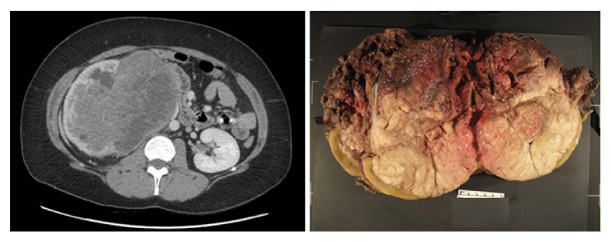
CT imaging shows a heterogeneous partially calcified enhancing solid mass of the right kidney and gross findings show a necrotic and friable soft tissue mass involving the distal ureteric margin and the renal vein.
